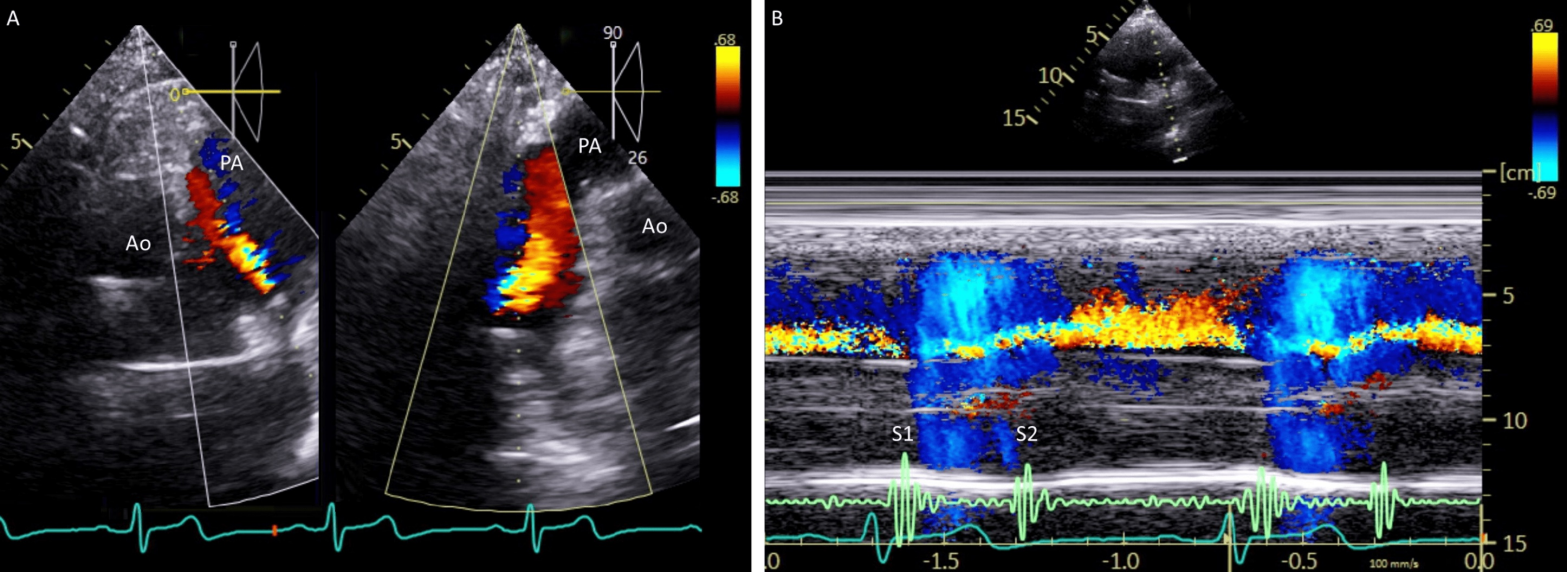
Echocardiography. Short-axis images (Panel A, left) at the level of the aorta (Ao) and its 90-degree rotated view (Panel A, right) show flow directed toward the pulmonary artery (PA). Note that, on the color M-mode (Panel B), the flow, which begins at the first heart sound (S1), persists through and beyond the second heart sound (S2).
